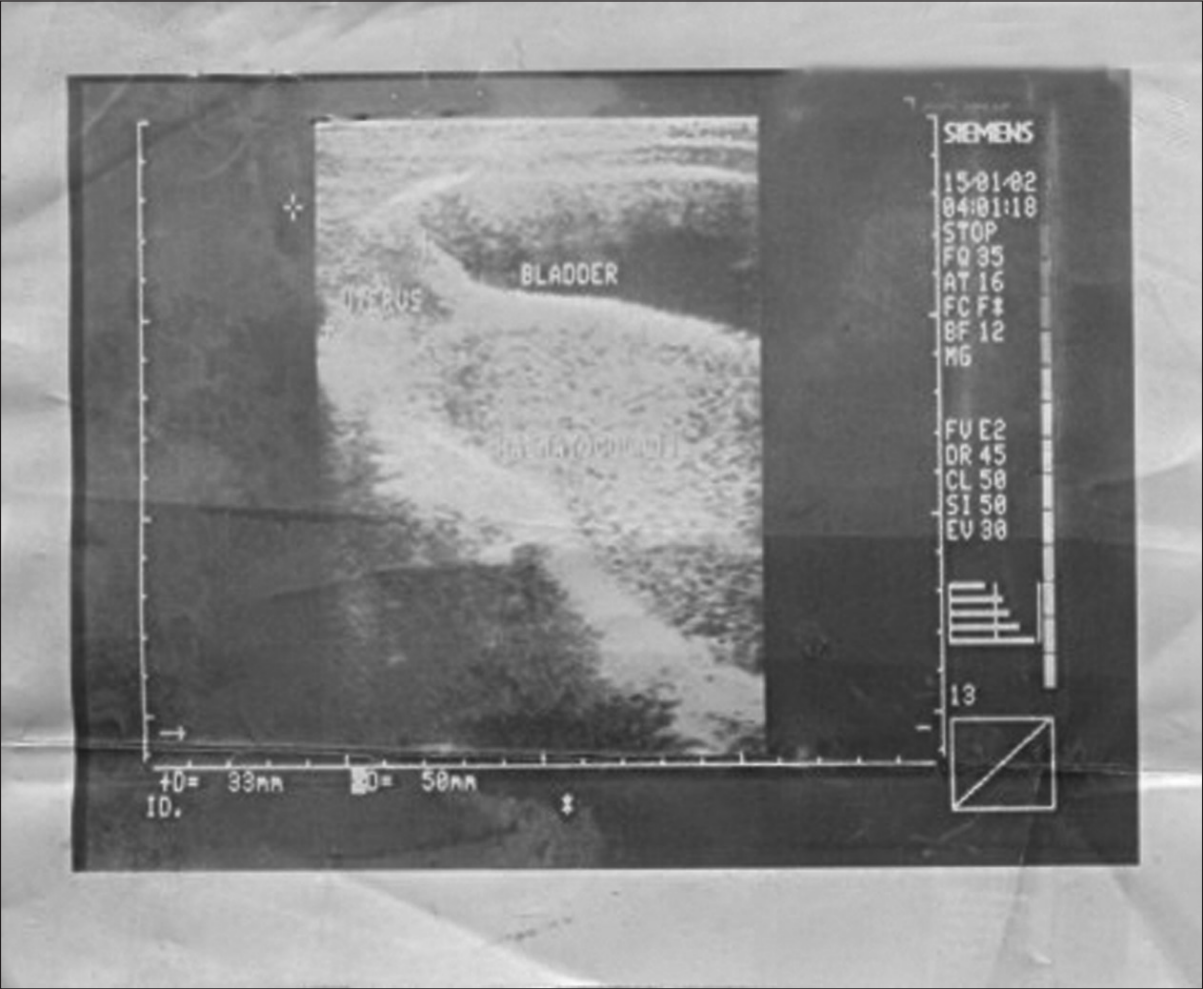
Ultrasound scan of the patient showing hematocolpos
